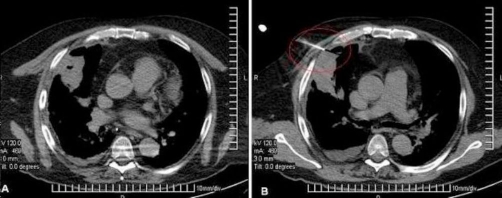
(A) Chest CT scan showing a cavitated right lung mass and right pleural effusion, (B) CT guided fine-needle lung biopsy.
